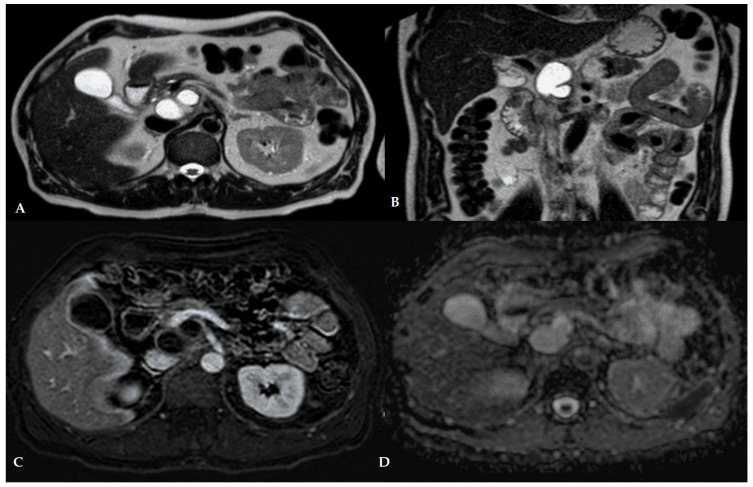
Axial (A) and coronal (B) T2-weighted MR images show a well-encapsulated, multiloculated fluid collection (arrows) with a homogeneously hyperintense T2 signal. No contrast enhancement is observed on post-contrast T1-weighted gradient-echo sequence (C), and no diffusion restriction is evident on the ADC map (D), consistent with a fluid content of serohematic/proteinaceous nature. The imaging features are compatible with a pseudocyst in a patient with a history of acute pancreatitis. The collection is located posterior to the pancreatic head and uncinate process, extending cranially into the porto-caval space.
